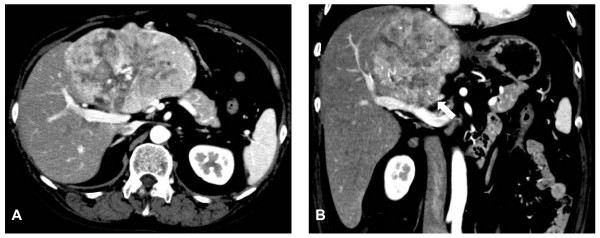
Contrast-enhanced computed tomography scans before surgical resection. (A) Arterial phase showed a large hypervascular lesion extending from the left lobe to the anterior segment of the right lobe. (B) The left branch of the portal vein was not identified as being involved in the tumor, invasion of the portal vein was suspicious (white arrow).
